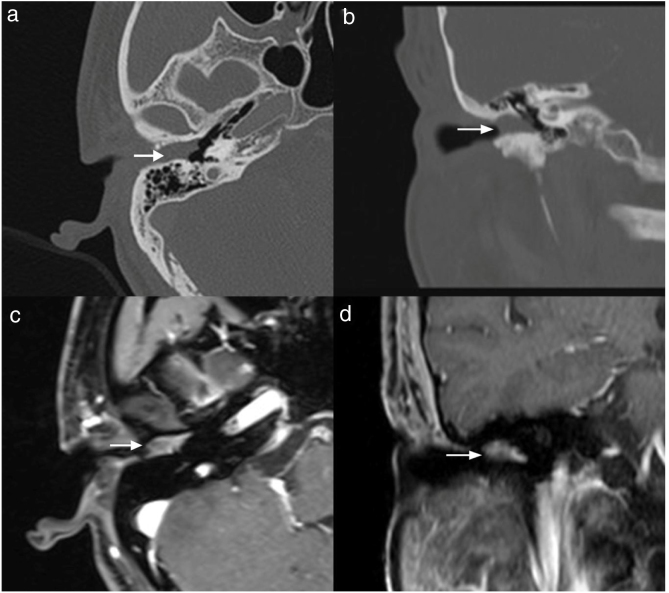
The temporal bone CT and MRI of Case 4. Temporal bone CT and MRI showed the lesion limited in EAC, without ossicular chain involved and bone erosion (A, axial; B, coronal). The mass was moderately enhanced with gadolinium administration on temporal bone MR (C, axial; B, coronal).
